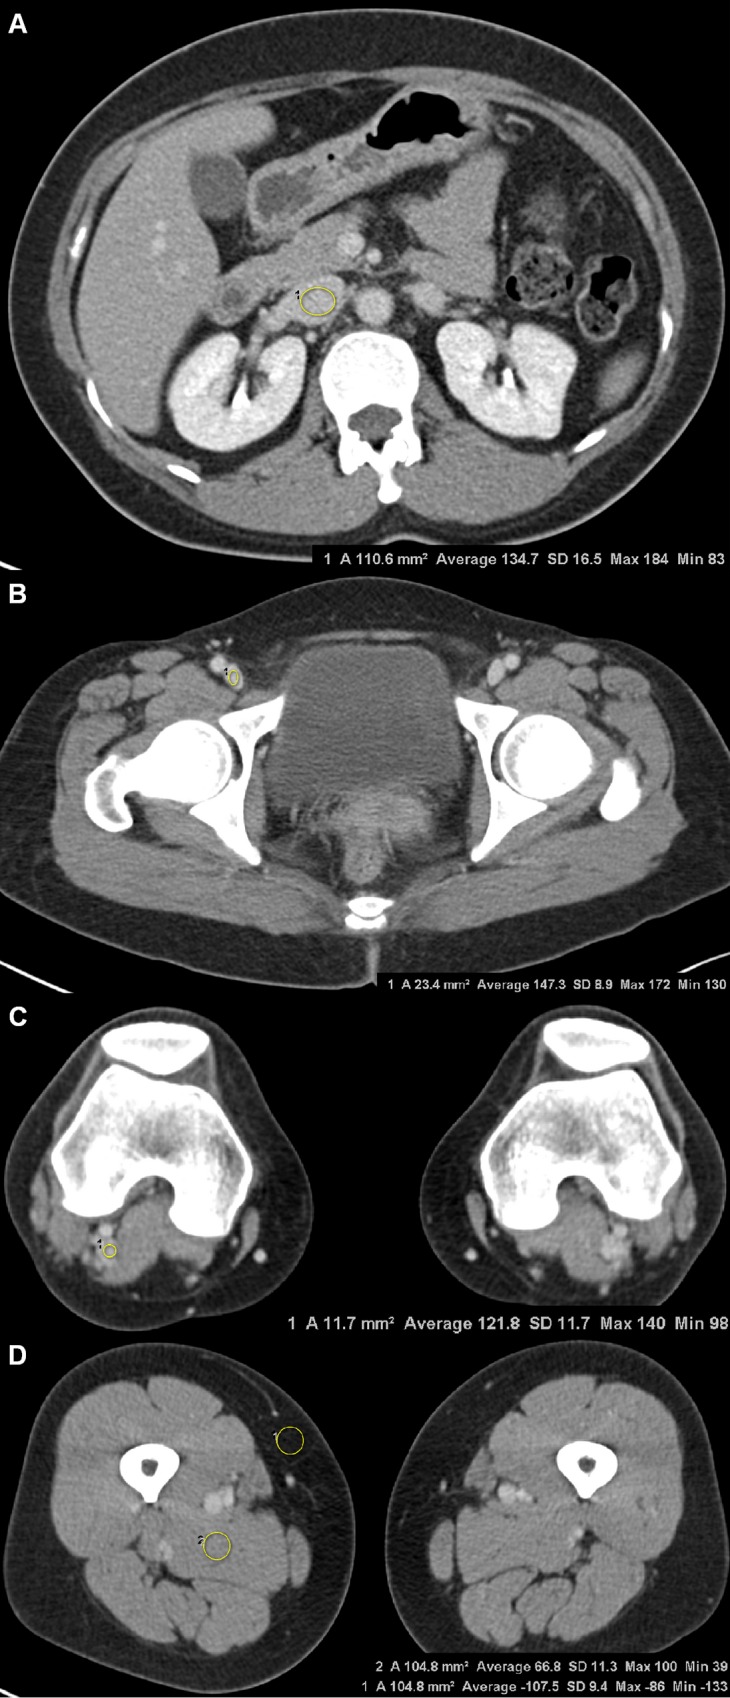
Axial CTV images obtained using 120 kVp and FBP in a 49-year-old woman with BMI 23.9. Attenuation value, vein-to-muscle contrast-to-noise ratio and signal-to-noise ratio in this patient were 134.7 HU, 7.2, and 8.2 in the IVC (a), 147.3 HU, 8.6, and 16.6 in the CFV (b), and 121.8 HU, 5.9 and 10.4 in the PV (c). Attenuation values of subcutaneous fat and muscle at the mid-thigh level were also measured using circular ROIs (d). The image quality was subjectively classified as score 4 (good) for the overall image quality and score 1 (optimal) for the image noise by the two readers. *CTV computed tomographic venography, FBP filtered back projection, BMI body mass index, HU Hounsfield unit, IVC inferior vena cava, CFV common femoral vein, PV popliteal vein, ROI region of interest.
